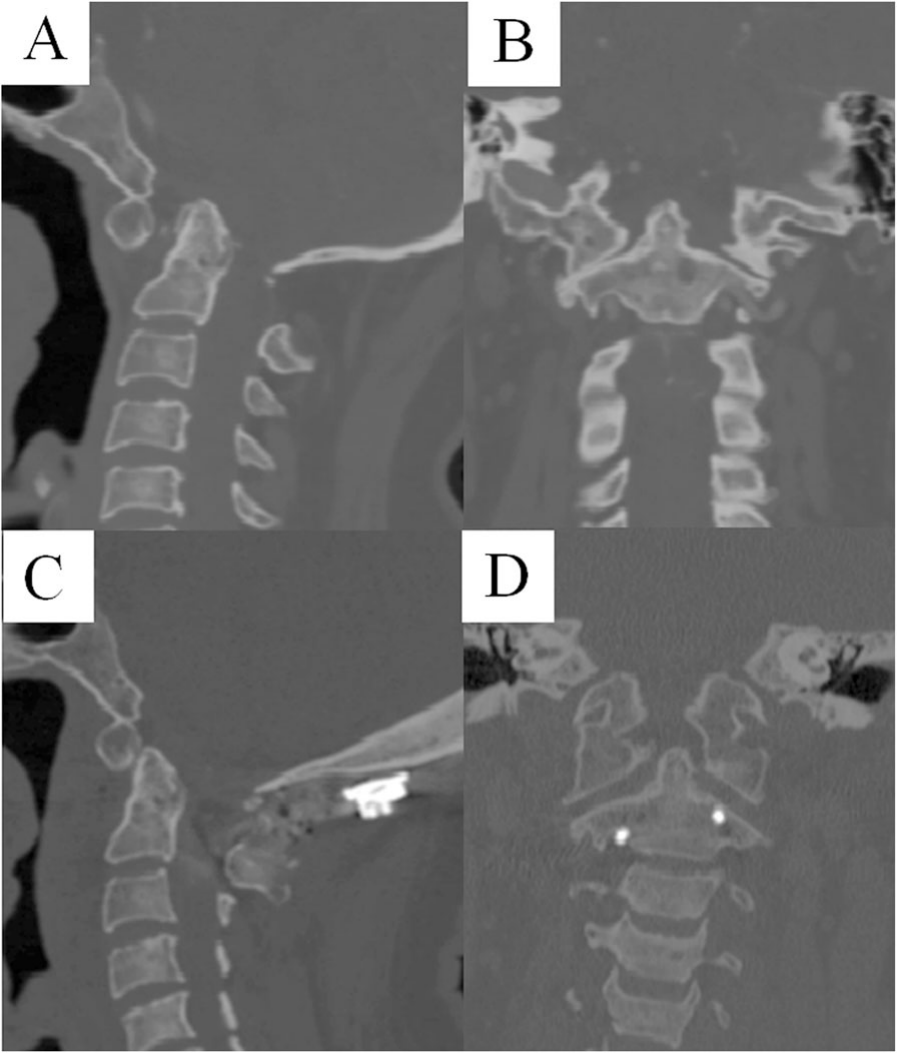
Case 26, a 57 years old female diagnosed as atlantoaxial dislocation and underwent retropharyngeal release and posterior C0–2 fixation. Pre-operative CT scan indicated atlantoaxial dislocation (a) and lateral atlantoaxial joints (b) in coronal reconstruction. Post-operative CT indicated anatomical reduction (c) and widening of lateral atlantoaxial joints (d)
